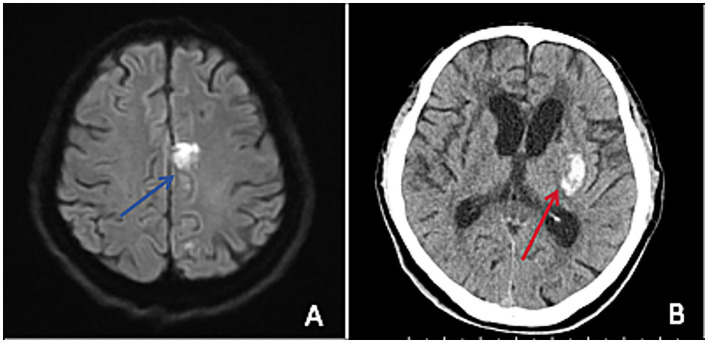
A case of HBC-3a hemorrhagic transformation following intravenous Tenecteplase thrombolysis. (A) Diffusion-weighted magnetic resonance imaging (DWI) demonstrates restricted diffusion consistent with acute cerebral infarction (blue arrows). (B) Non-contrast head computed tomography (CT) shows a hyperdense intraparenchymal hemorrhage corresponding to hemorrhagic transformation (red arrows).
